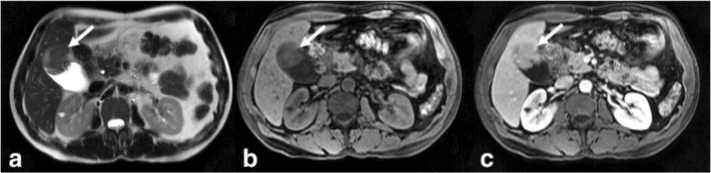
Gall bladder carcinoma arising from the fundus of the gall bladder (arrow) seen as an iso to hyperintense mass on T2-weighted (a) and hypointense mass on T1-weighted (b) images and shows enhancement on post gadolinium enhanced image (c).
